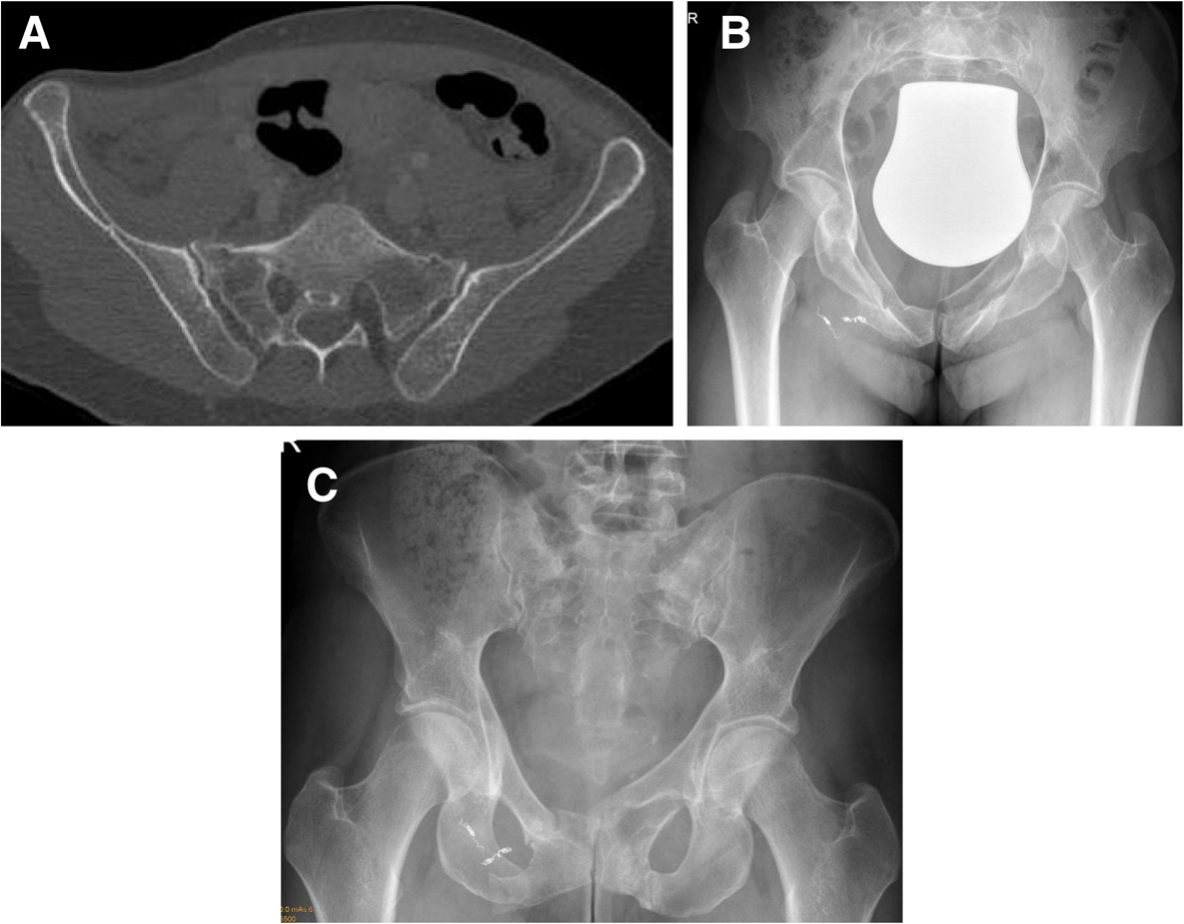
Clinical example for non-operative therapy of a lateral compression fracture. a shows the initial CT-scanning with sacral fractures on both sides. b shows the pubic rami fractures and the coils after embolization. c shows consolidation after 6 weeks. The patient presented with full weight bearing and without pain
